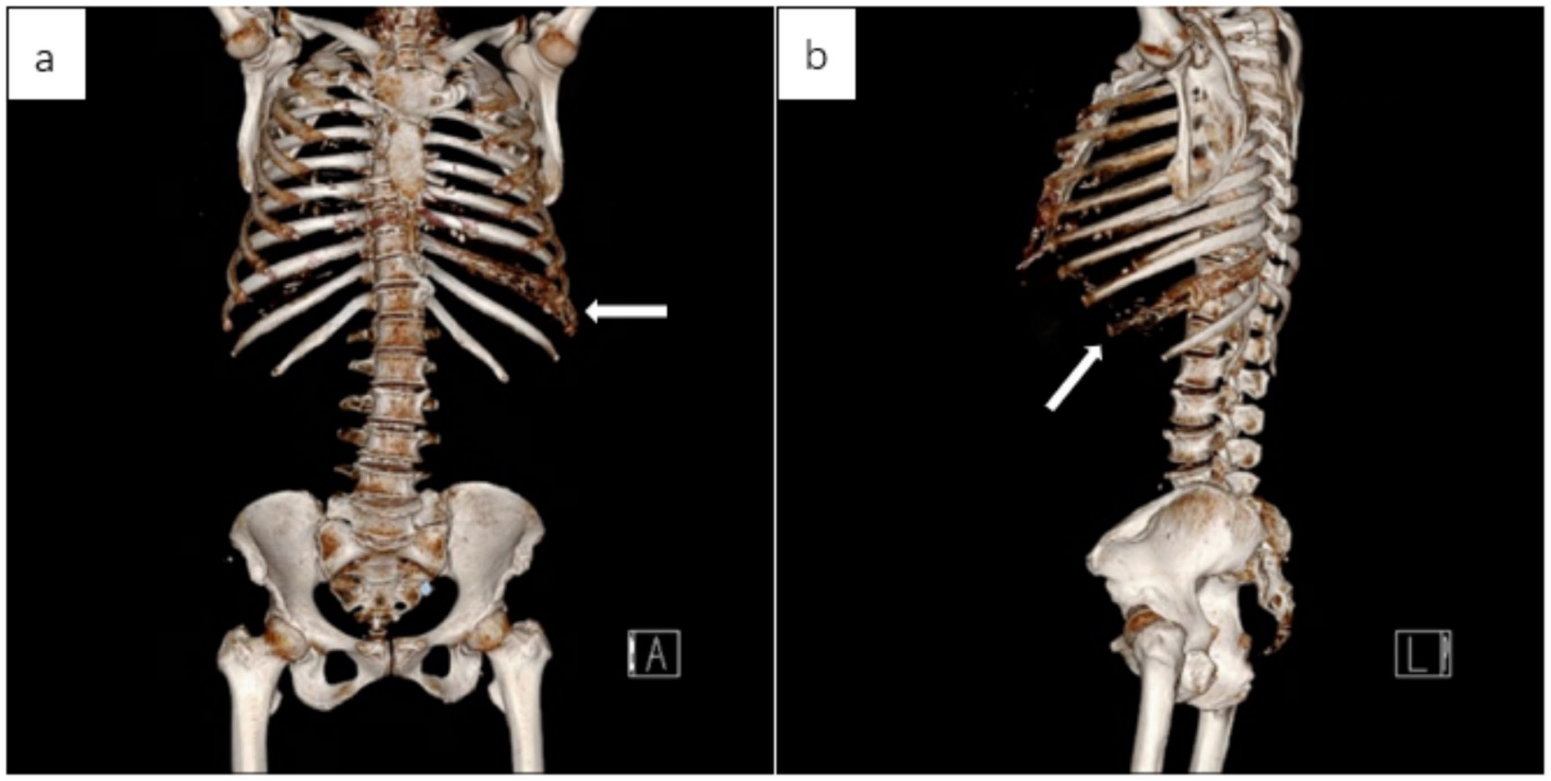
CT (bone window) of the chest, abdomen, and pelvis demonstrates an expansile osteolytic lesion of the left ninth rib: (a) Coronal reformatted image and (b) sagittal reformatted image.
